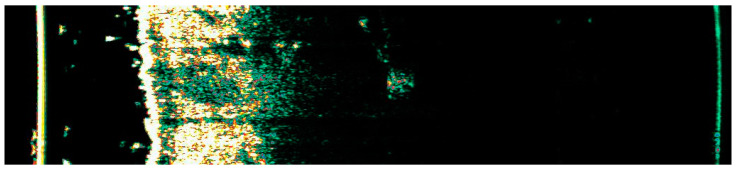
Ultrasonographic image of BCC on the upper arm.
